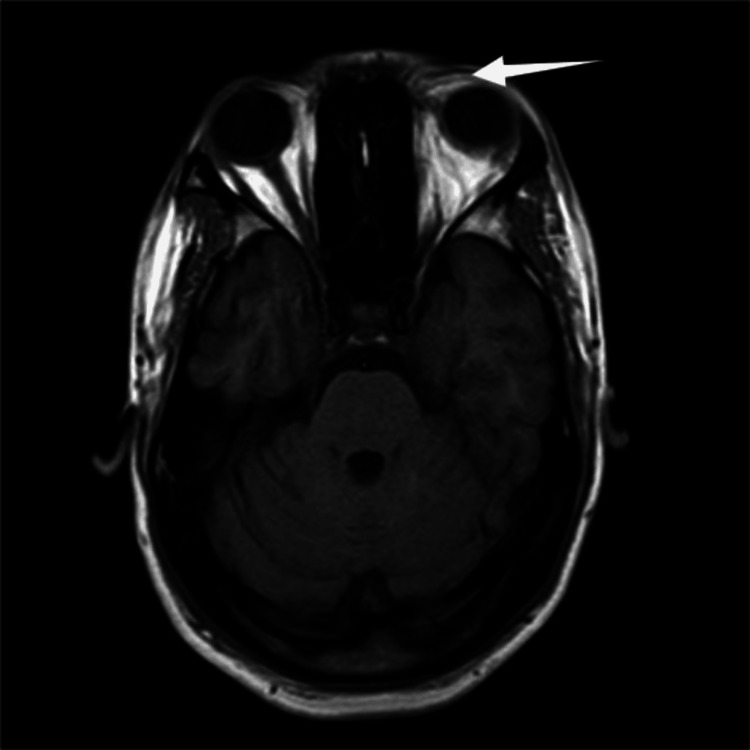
MRI T2 FLAIR axial image of the orbit, showing mild soft-tissue hyperintensities (white arrow) in the medial aspect of the preseptal region of the left orbit, suggestive of preseptal edema MRI: magnetic resonance imaging; FLAIR: fluid-attenuated inversion recovery
